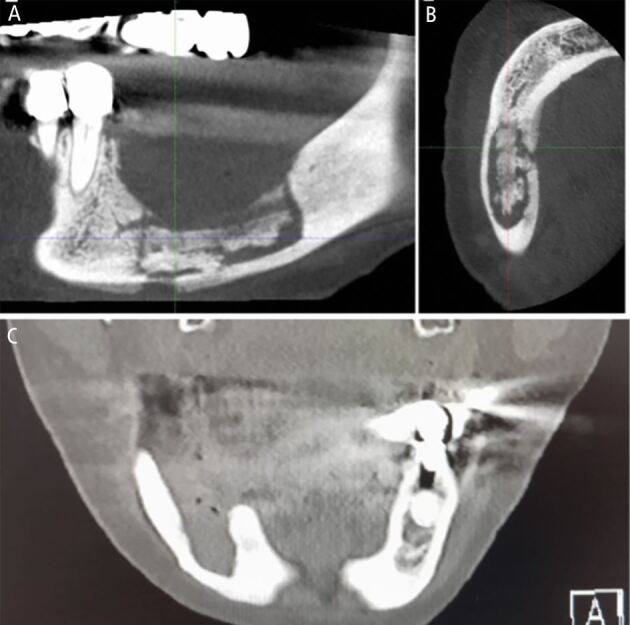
Case 3. (A) Pre-operative CBCT sagittal view of MRONJ. (B) Pre-operative CBCT axial view of MRONJ. (C) Most recent post-operative coronal CT imaging
